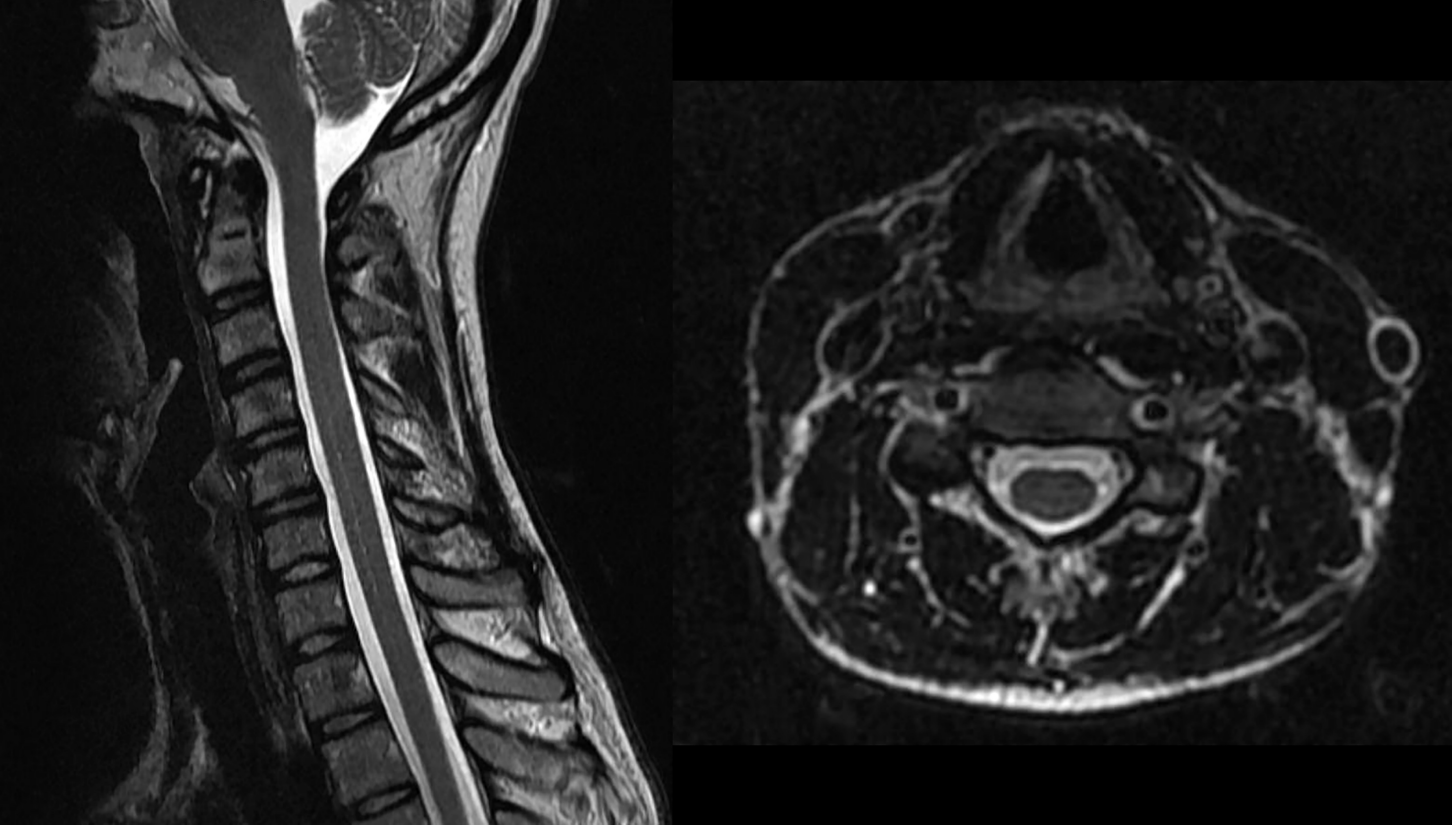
Sagittal and axial T2-weighted images demonstrate normal cervical cord signal intensity and morphology Note that bilateral vertebral artery flow voids are normal.
